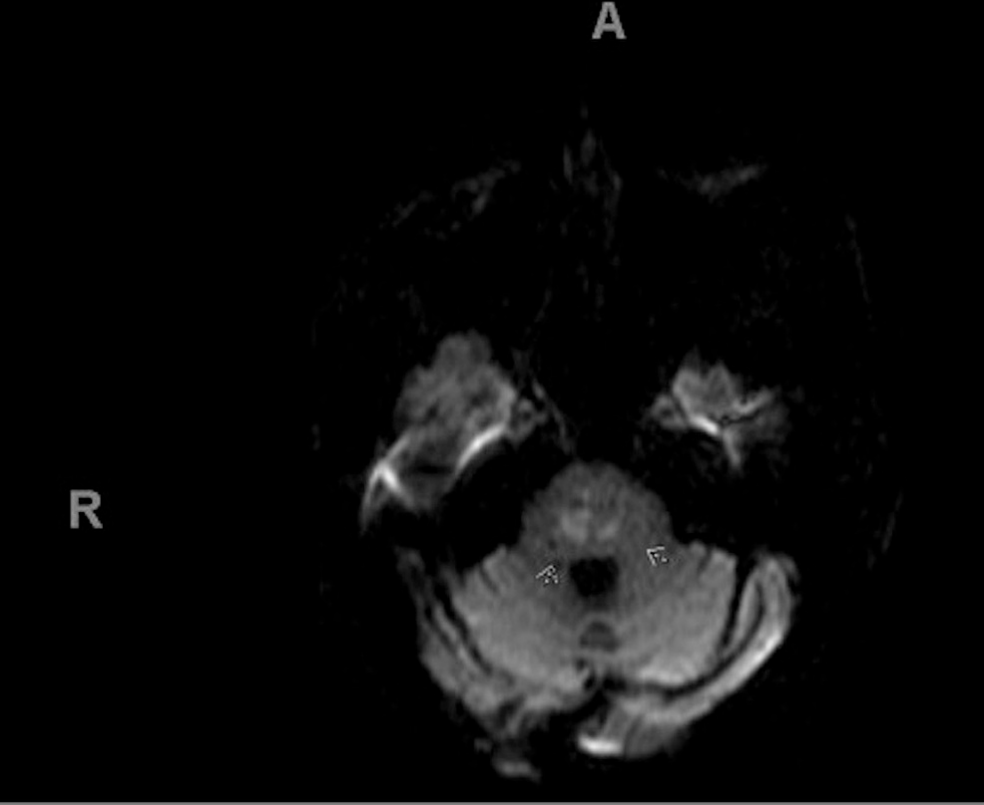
T2-weighted MRI of the brain, arrows showing hyperintense lesions in the central portion of the pons.
